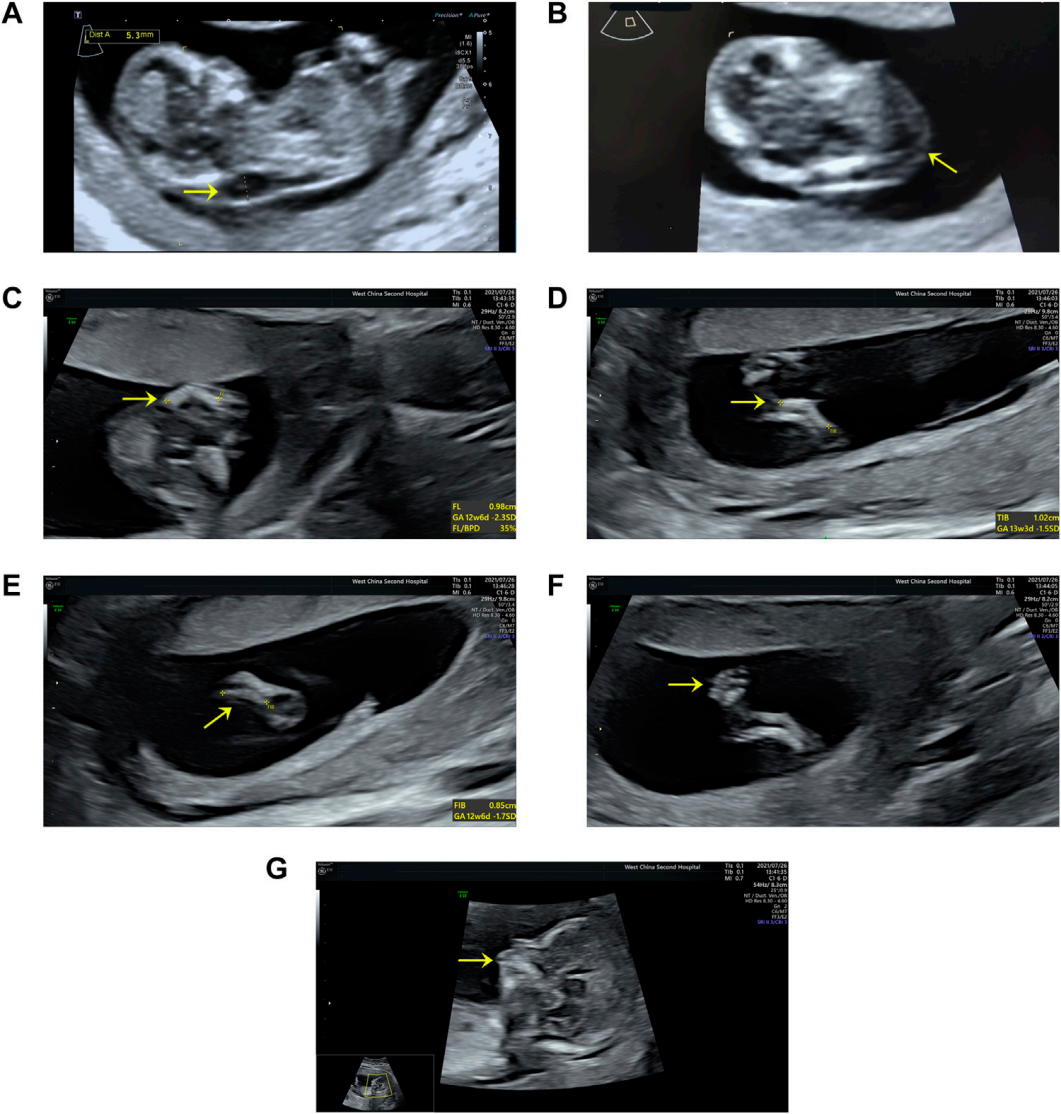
Ultrasound findings: (A) Cystic hygroma measuring 18 × 6 × 14 mm (CRL:60.9 mm) at the mid-sagittal plane. (B) Cystic hygroma in a transverse view. (C) Short and bowed femurs (<first centile). (D) Sagittal anterior angulated tibiae (fifth centile). (E) Sagittal anterior angulated fibula (fifth centile). (F) Unilateral equinovarus clubfoot. (G) Micrognathia.
